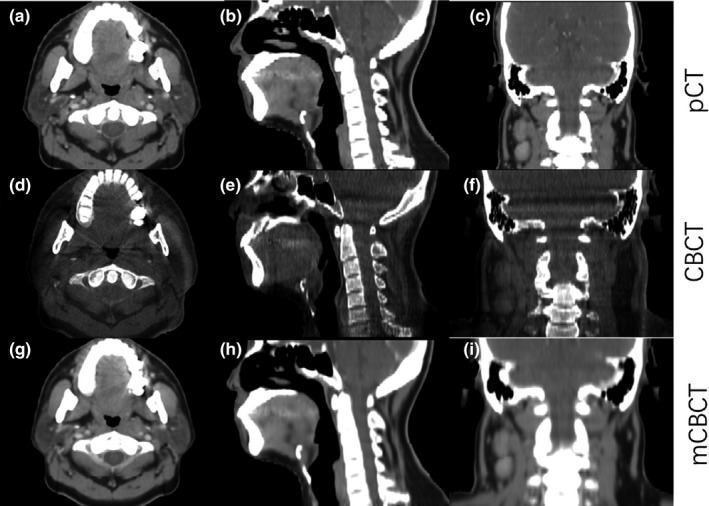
An example of the transverse plane, sagittal plane, and coronal plane of planning CT, CBCT, and mCBCT.
